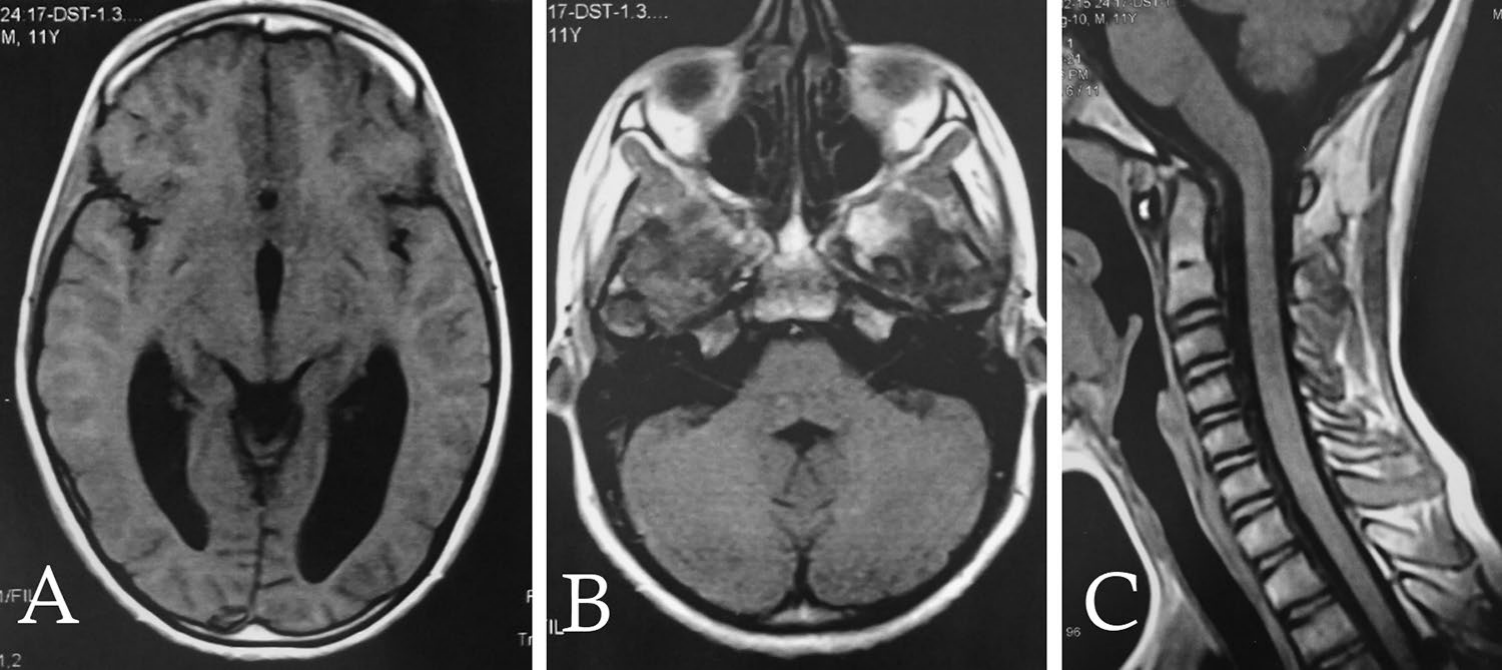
A 6-month follow-up MRI brain and cervical spine showing A marked regress of hydrocephalus, B complete resorption of the posterior fossa SDH, and C complete resorption of the clival, retro-cerebellar, and cervical SDH
